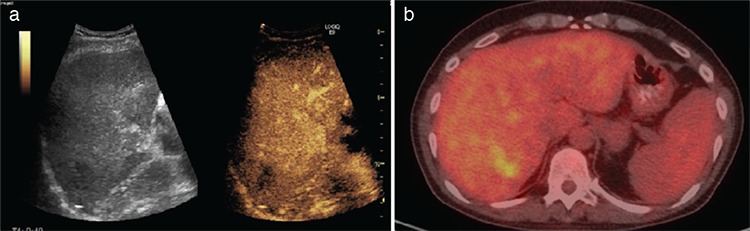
Results from the restaging PET/CT and CEUS of the same patient performed 2 months after the RFA were discrepant. The CEUS showed complete necrosis, interpreted as complete response, while on PET/CT there was a persistent metabolically active lesion with partial reduction of the size and activity (SUV_max_ 3), interpreted as partial response. a) CEUS, post-treatment image showing complete necrosis, b) fused axial image on the level of the 7^th^ liver segment, post-treatment, showing a persistent metabolically active lesion with partial reduction of the size and activity PET/CT: Positron emission tomography/computed tomography, CEUS: Contrast-enhanced ultrasound examination, RFA: Radiofrequency ablation, SUV_max_: Maximum standard uptake value
